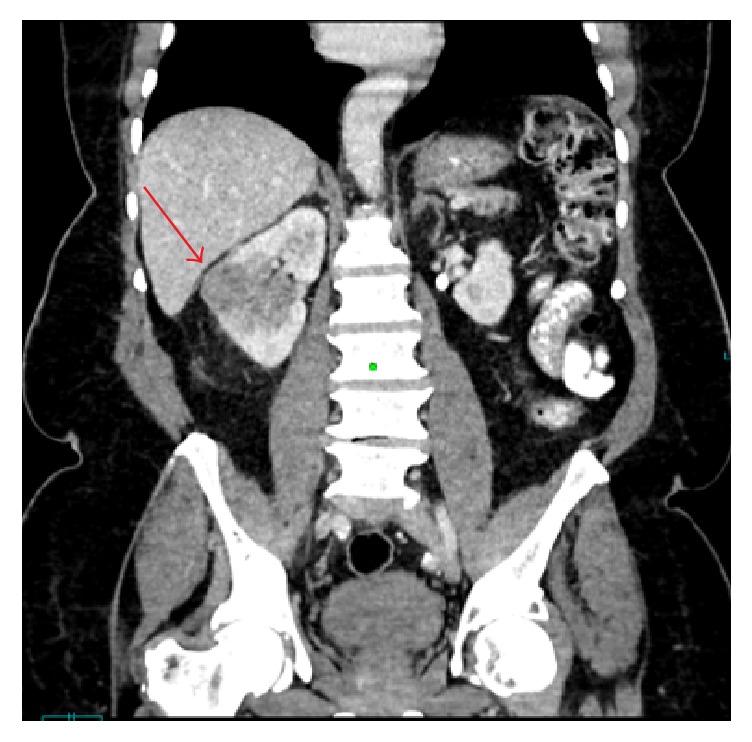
Computed tomography with contrast (CT) coronal image demonstrates a heterogeneously enhancing hypodense right renal mid pole mass (red arrow) occupying the renal cortex and renal pelvis with mild surrounding fat stranding.
